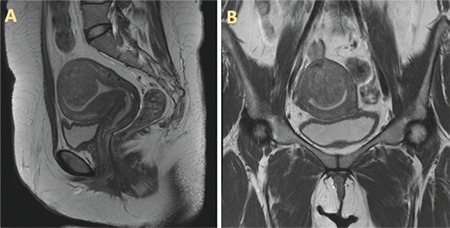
Pelvic magnetic resonance imaging (MRI). (A) Sagittal and (B) coronal pelvic MRI showed a sub-mucosal, posterior fundal uterine leiomyoma measuring 4x4x4.5 cm
